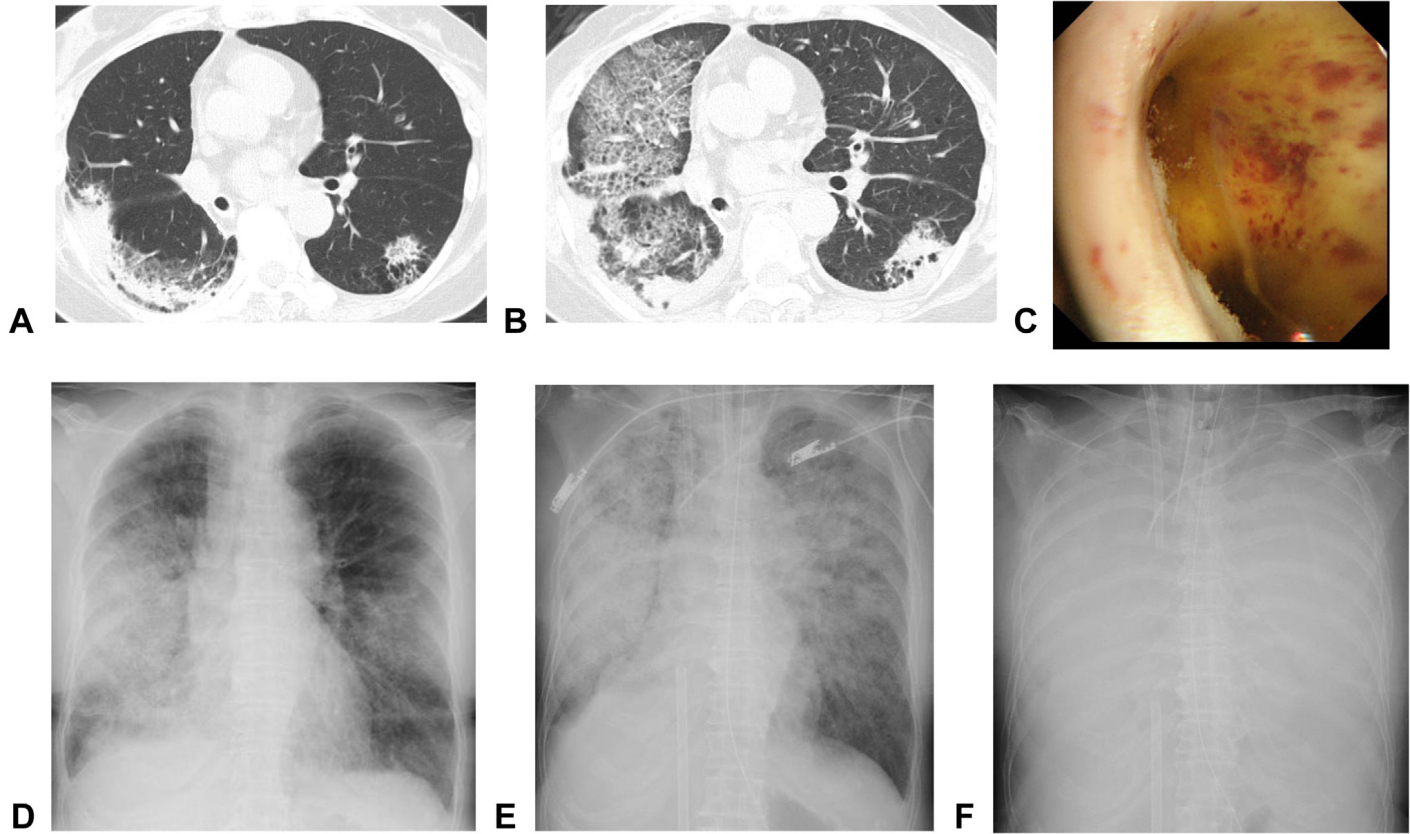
(*A*) Chest CT on day 43 revealed infiltrative shadows with contraction tendency in both lung lobes. (*B*) Chest CT on day 87 revealed extensive consolidation and ground-glass opacity in both lung lobes. (*C*) The bronchial lumen at the junction of the right upper lobe branch and the middle bronchial trunk was filled with yellow fluid. (*D*) Chest radiograph on day 87 (time of admission) revealed consolidation in both lung fields. (*E)* Chest radiograph on day 87 (8 hours after admission) revealed rapid deterioration of consolidation in both lung fields. (*F*) Chest radiograph on day 87 (16 hours after admission) revealed a complete loss of permeability in both lung fields. CT, computed tomography.
